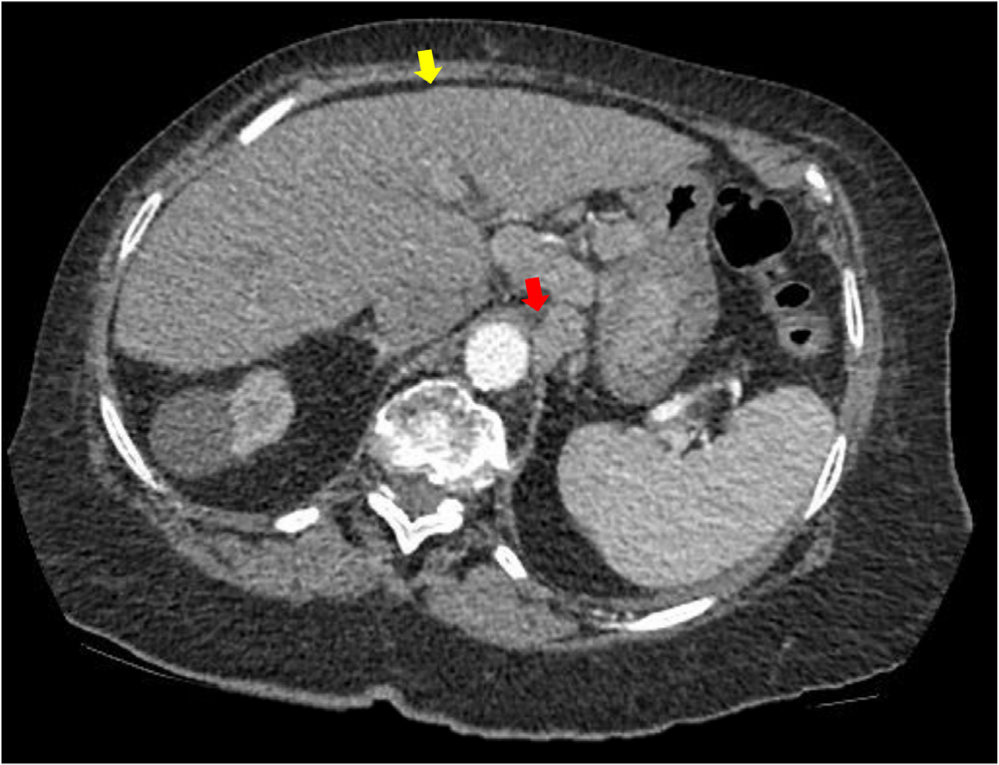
Axial postcontrast CT demonstrating the primary gastroesophageal mass indicated by the red arrow and nodular hepatic contours consistent with a cirrhosis-like appearance indicated by the yellow arrow.
